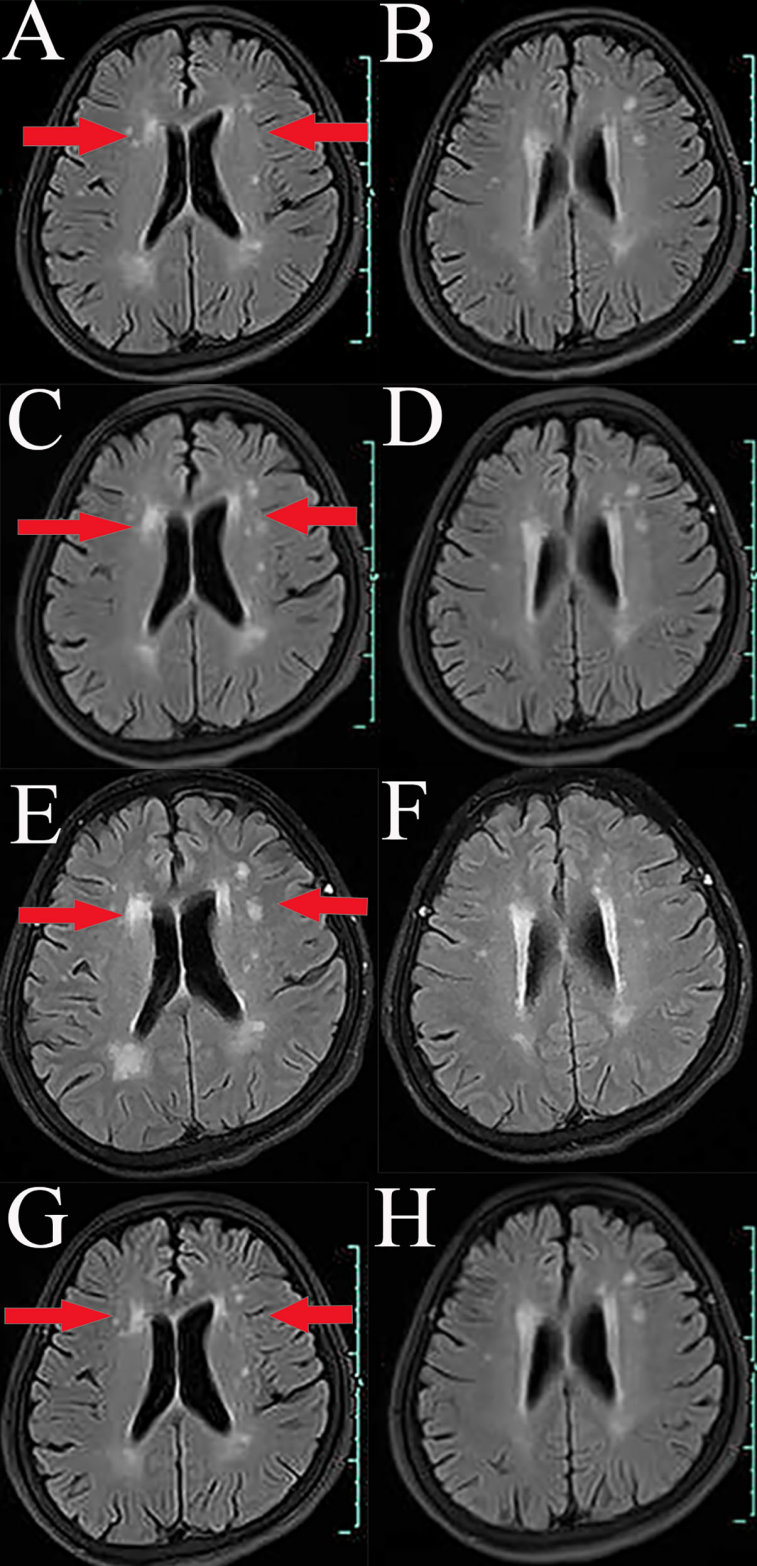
Variations of occupation on brain areas by MRI images during the treatment (red arrowheads). (A, B), February, 2022. (C, D), February, 2023. (E, F), April, 2024. (G, H), July, 2024.
